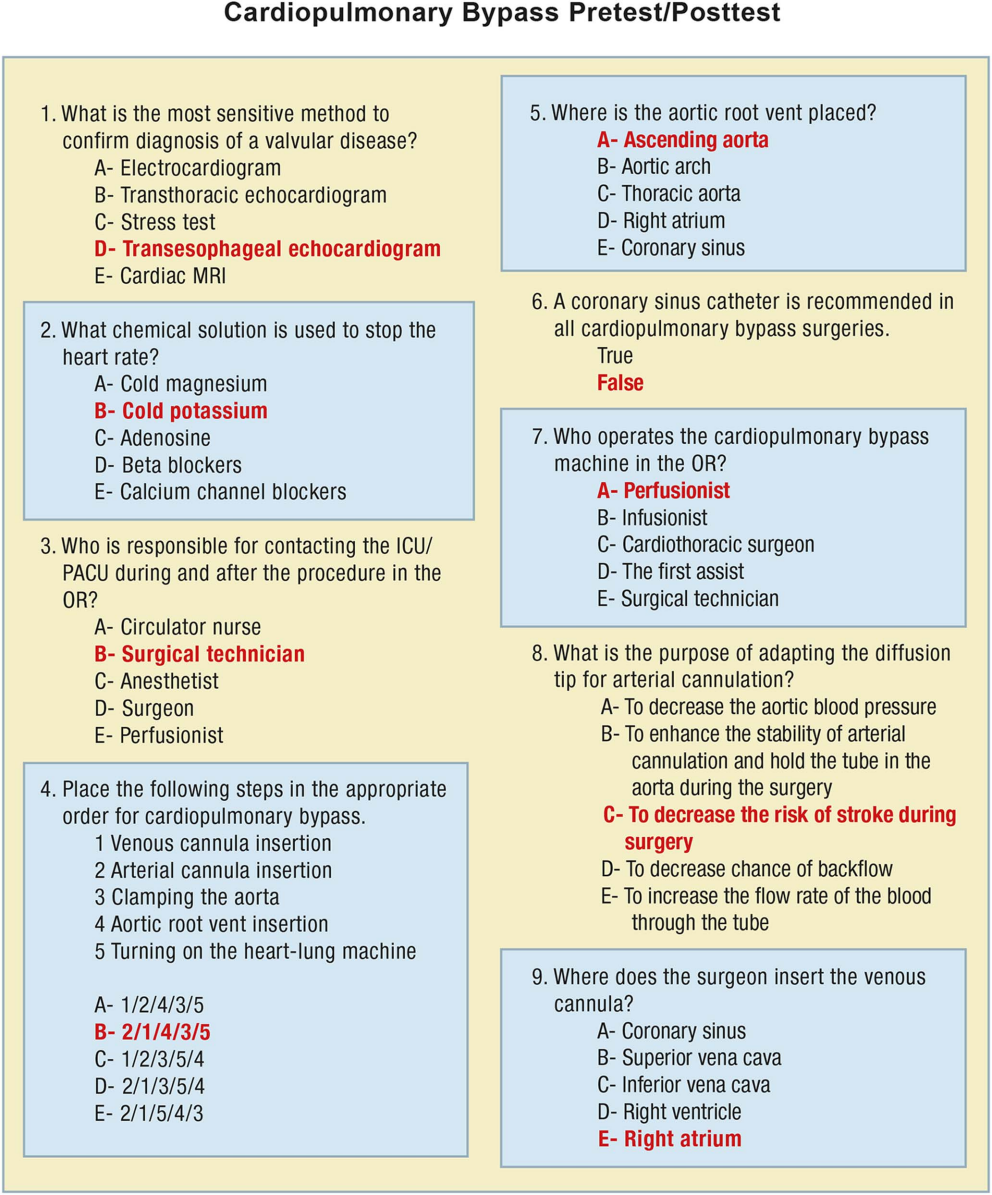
Cardiopulmonary bypass video pretest/posttest with correct answers in boldface. ICU, intensive care unit; MRI, magnetic resonance imaging; OR, operating room; PACU, postanesthesia care unit.
